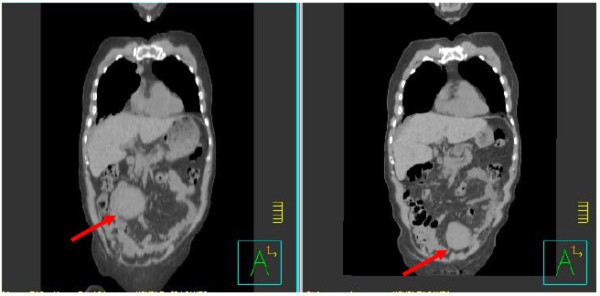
Coronal treatment-planning computed tomography scans obtained five days apart showing the abdominal tumor in two distinctly different locations.
